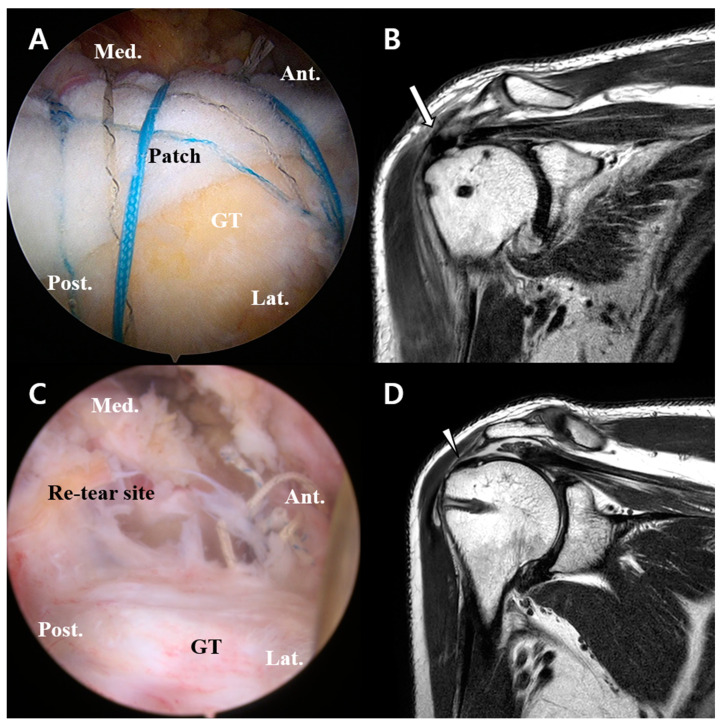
Arthroscopic views and MRIs of re-tear patient. (A) Patch augmentation using human allograft for a large rotator cuff tear. (B) Postoperative MRI shows a well-maintained patch graft covering GT footprint (arrow). (C) Second-look arthroscopic view. A re-tear can be identified, and GT-covering fibrotic tissue can also be seen. (D) A T2-weighted coronal image of the right shoulder from a pre-second-operation MRI reveals a major discontinuity (a Sugaya Grade V re-tear) of the supraspinatus tendon. A fibrosis remnant of the allograft patch covers the GT footprint area (arrowhead) despite the re-tear. MRI—magnetic resonance imaging; GT—greater tuberosity.
